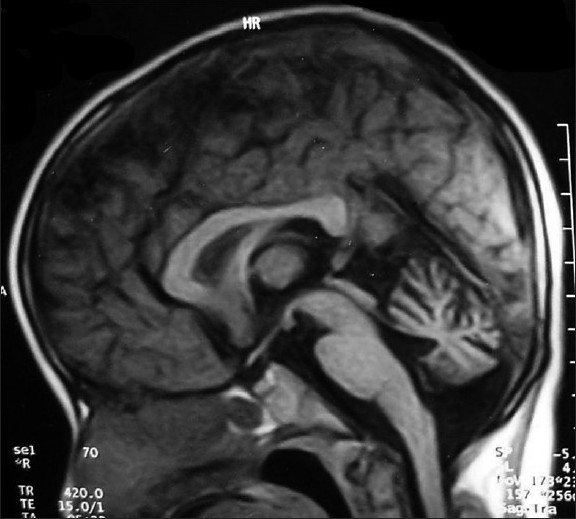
MRI Brain showing cerebellar atrophy.
